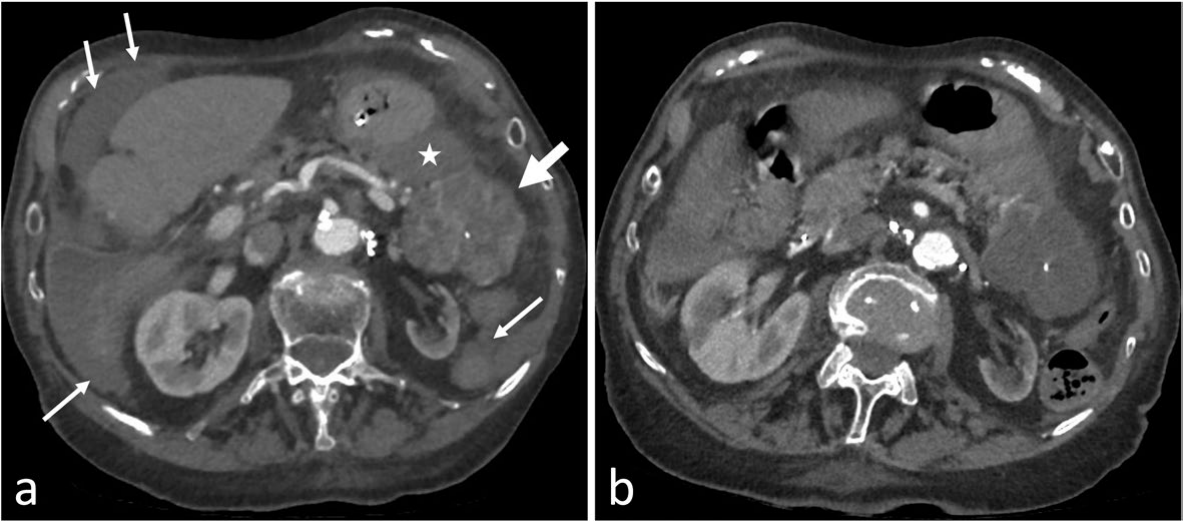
Axial CT-scan images, at the late arterial phase, before (a) and after (b) embolization procedure. a Initial CT-scan revealed a hematoma (star) next to the pancreatic tumor (thick arrow) and diffuse hemoperitoneum (thin arrows). Note the hypervascularisation of the tumor septa and the central calcification, both characteristic of serous cystadenoma. The lesion doubled in size in thirteen years (66 mm vs 31 mm). b CT-scan 2 days after embolization confirmed the disappearance of the hypervascularization of the tumor, with no signs of further bleeding
